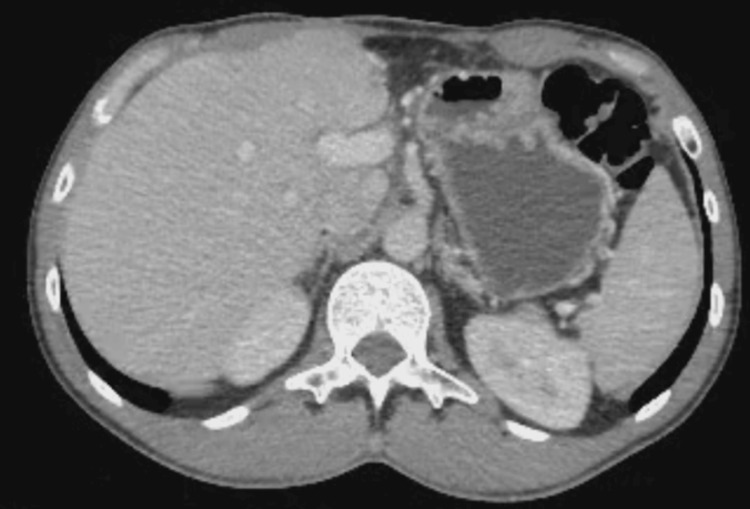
Follow-up contrast-enhanced CT after hepatic resection and immunotherapy Abdominal contrast-enhanced CT scan performed during follow-up after hepatic resection and nivolumab therapy, demonstrating no radiological evidence of residual or recurrent hepatic metastatic disease.
